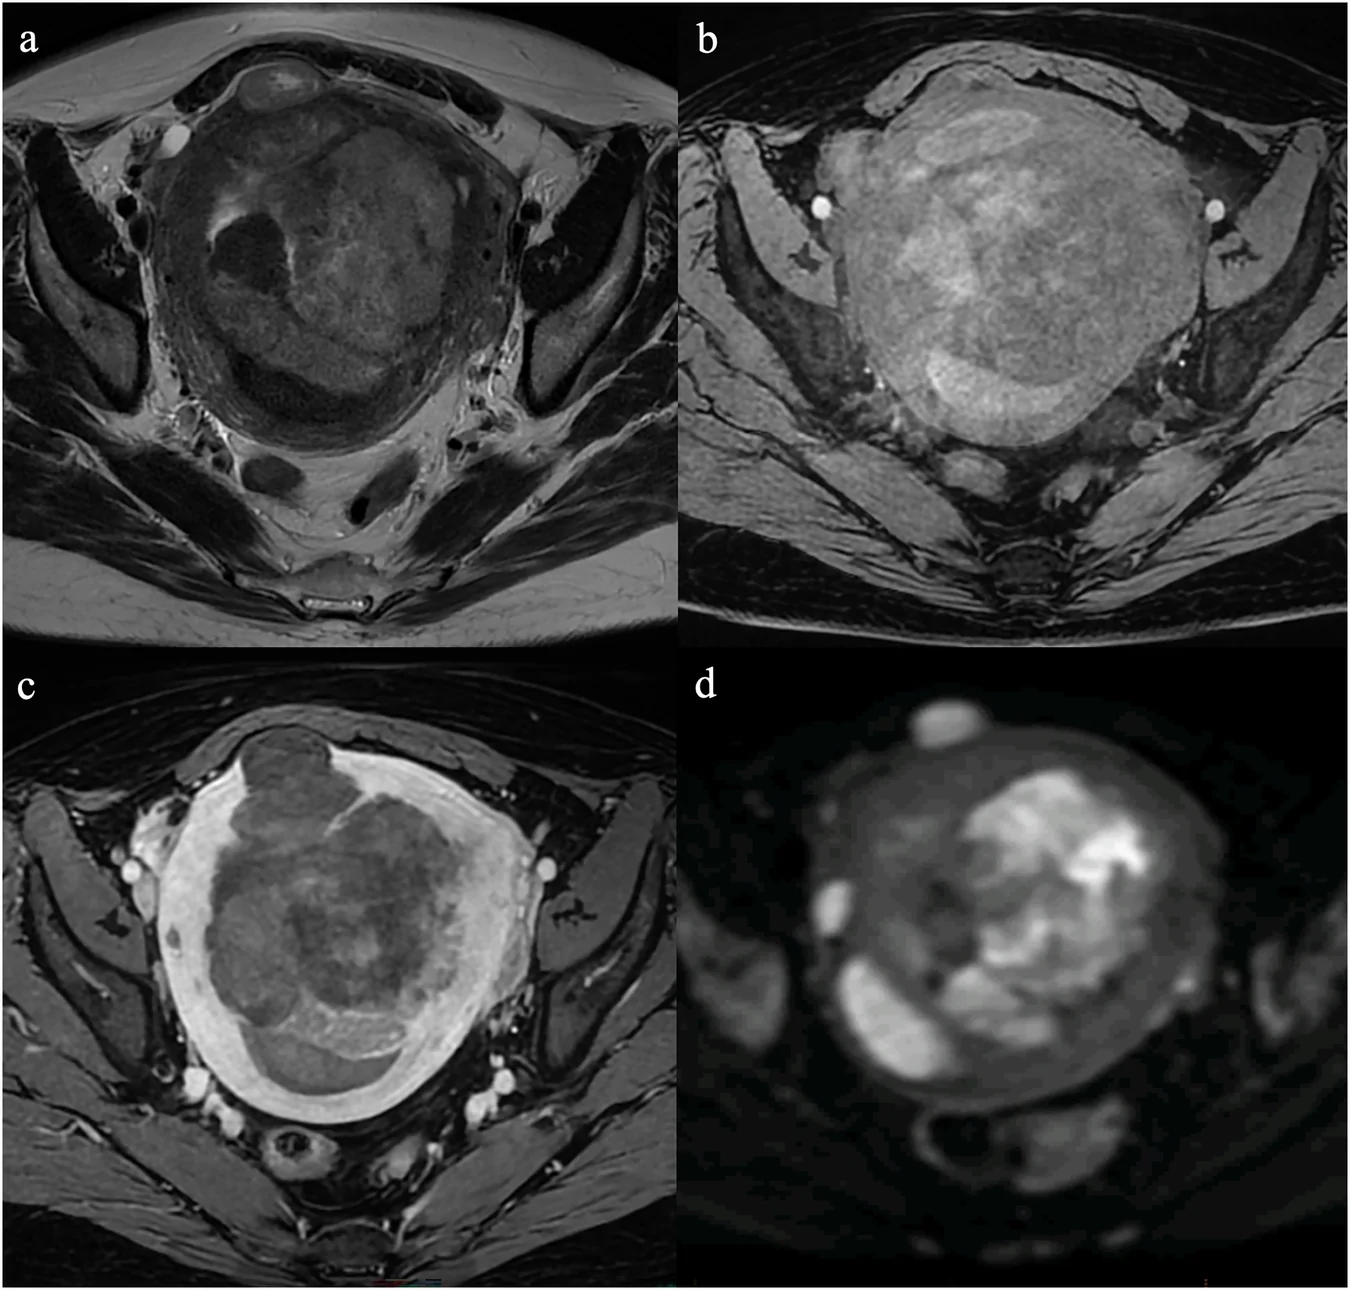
Leiomyosarcoma. a Axial T2-weighted sequence; b axial water-only image from a T1-weighted Dixon sequence; c axial postcontrast fat-saturated T1-weighted sequence; d axial DWI sequence. Image is showing a heterogenous ill-defined myometrial lesion with intermediate T2W signal, spontaneous T1W signal corresponding to hemorrhage. Postcontrast imaging demonstrates large necrotic areas and infiltrative peripheral parts with relatively high DWI signal. ADC map (not shown) demonstrated values as low as 0.8 × 10⁻³ mm²/s in the enhancing component. As the patient was postmenopausal, the lesion was classified as score 5 [7]
